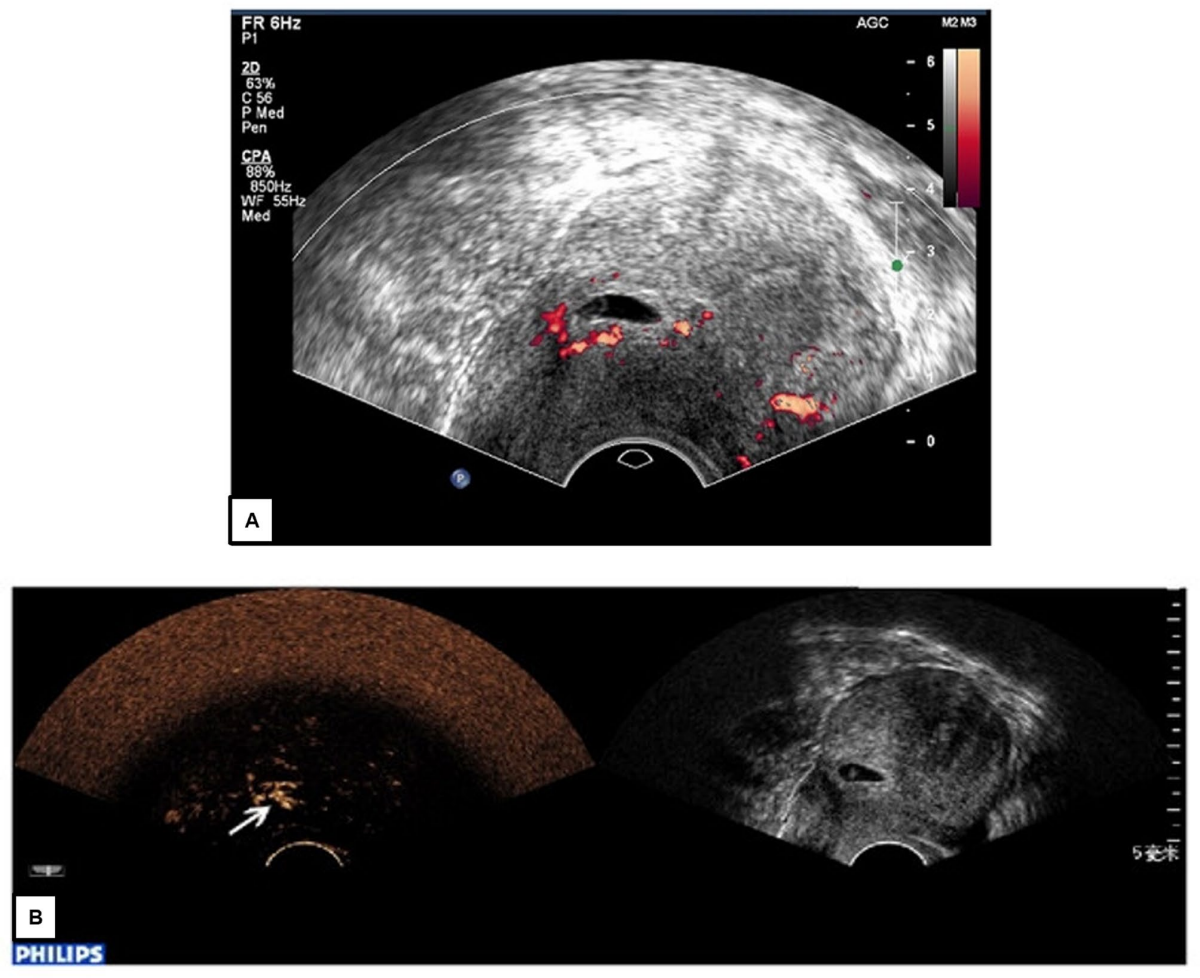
Clinical application of CEUS in pregnancy. A Cesarean Scar Pregnancy initially misdiagnosed as intrauterine pregnancy on conventional ultrasound. B Contrast-enhanced ultrasound of the same patient showing blood supply to the gestational sac directly from the uterine scar (arrow), establishing correct diagnosis. Adapted from Xiong et al. [41]
